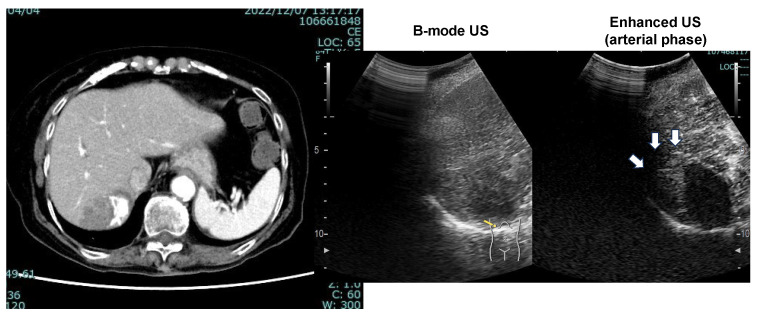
CT image (arterial phase) and enhanced US after 12 courses of ATZ/BEV therapy. Left panel: CT image showing the disappearance of lipiodol and the appearance of arterial flow on the right side of the HCC. Right panel: Contrast-enhanced ultrasound revealing arterial flow in the arterial phase and a defect in the Kupffer phase.
